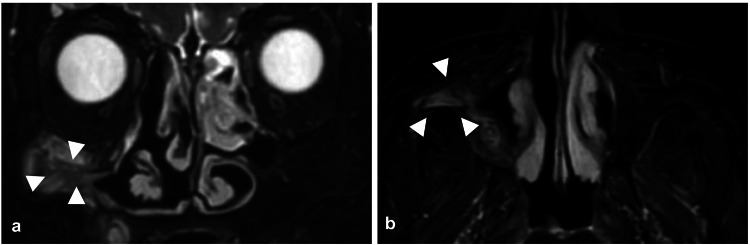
Postoperative MRI T2-weighted images are shown. The high-intensity area indicating postoperative maxillary cyst (POMC), observed preoperatively, disappeared. White arrowheads indicate the cyst. (a) shows a coronal slice and (b) shows an axial slice.
